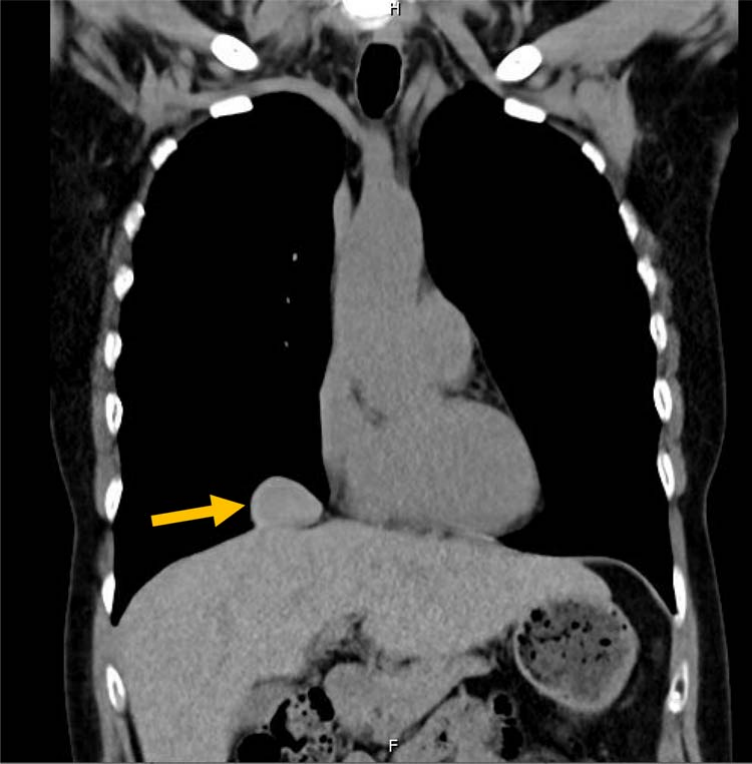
Larger lung nodule measuring 1.5 cm × 2.8 cm on CT scan.
